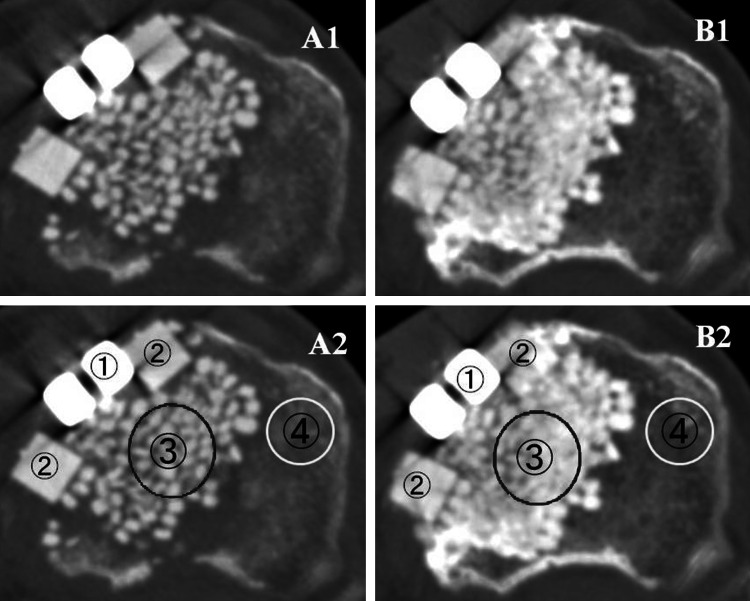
Case 5: 63-year old woman. Computed tomography (CT) images showing the centre of the osteotomy plane at 1 month (A1, A2) and 12 months (B1, B2). The mean CT values (HU) of the area implanted with the novel unidirectional porous hydroxyapatite block (➁), the area implanted with granules (➂), hinged-cancellous bone (➃) changed from 950, 662, and 172, to 798, 951, and 186, respectively. ➀ indicates the area of the metal plate
